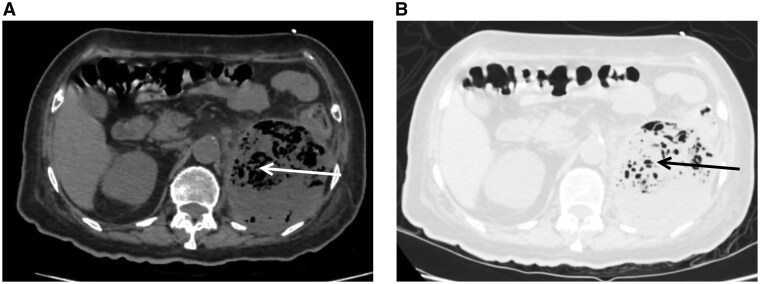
Axial non-contrast CT images (A-Soft window) and (B-Lung window) demonstrating extensive gas within the left renal parenchyma (arrows) consistent with emphysematous pyelonephritis.
